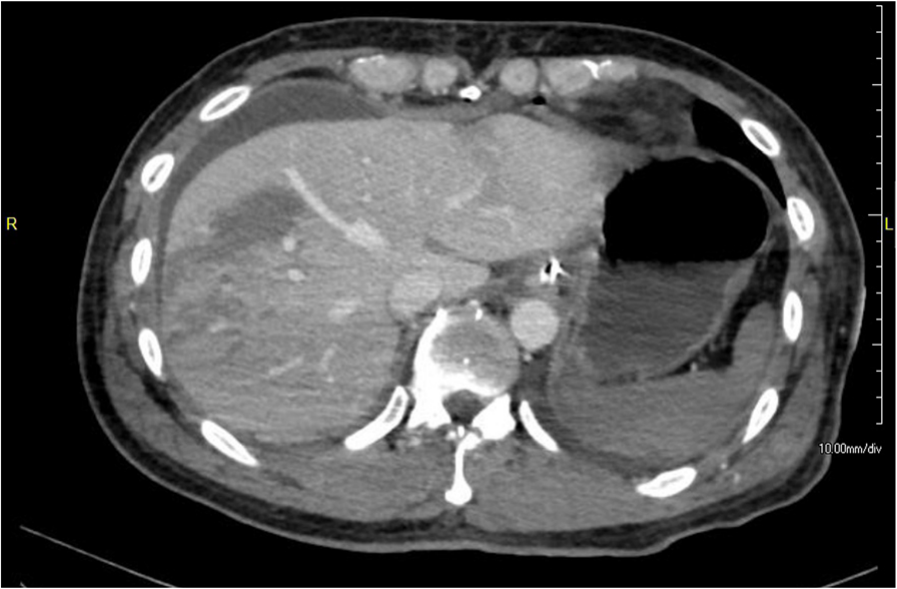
Multidetector CT 1 day after the PD showing widespread liver ischemia (the *arrow* shows the CA and the *arrow head* shows the SMA)
